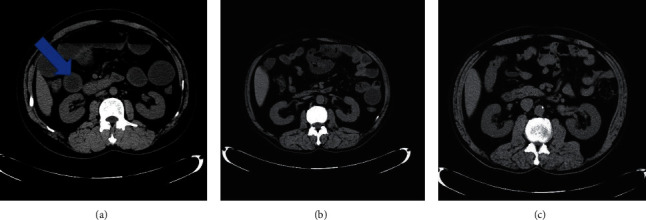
CT image of the largest diameter of the proximal colon: before surgery (a); 3 days after surgery (b); 7 days after surgery (c). Note that the arrow indicates the location of the patient's intestinal obstruction.
